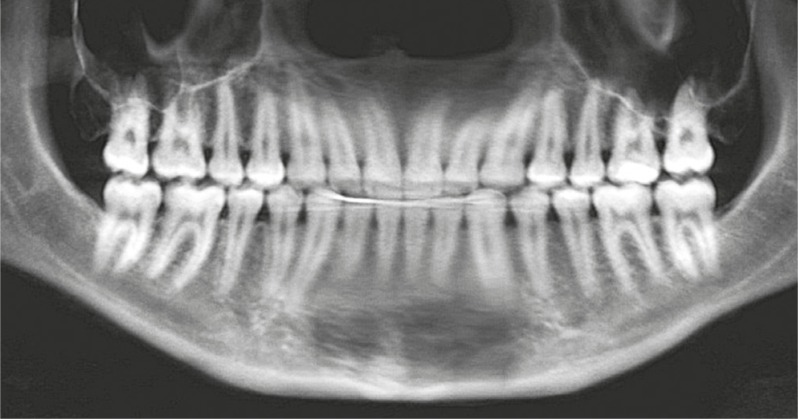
Final panoramic radiograph.
